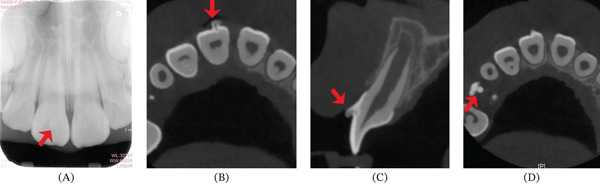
Serial radiographs. (A) Intraoral periapical radiograph of a faintly visible o‐shape opaque image (red arrow) superimposed with the middle crown of the Tooth #11. (B, C) CBCT images with the extra cusp‐like structure probably containing enamel and dentin component according to image density. (B) Axial plane: a small hollow tubercle projected from the labial surface of the Tooth #11 (red arrow); (C) sagittal plane: a small projection with fissure parallel to the labial surface at the junction of crown cervical 1/3 and middle 1/3(red arrow). (D) Red arrow refers to an abnormal central cusp on occlusal surface of the Tooth #15.
